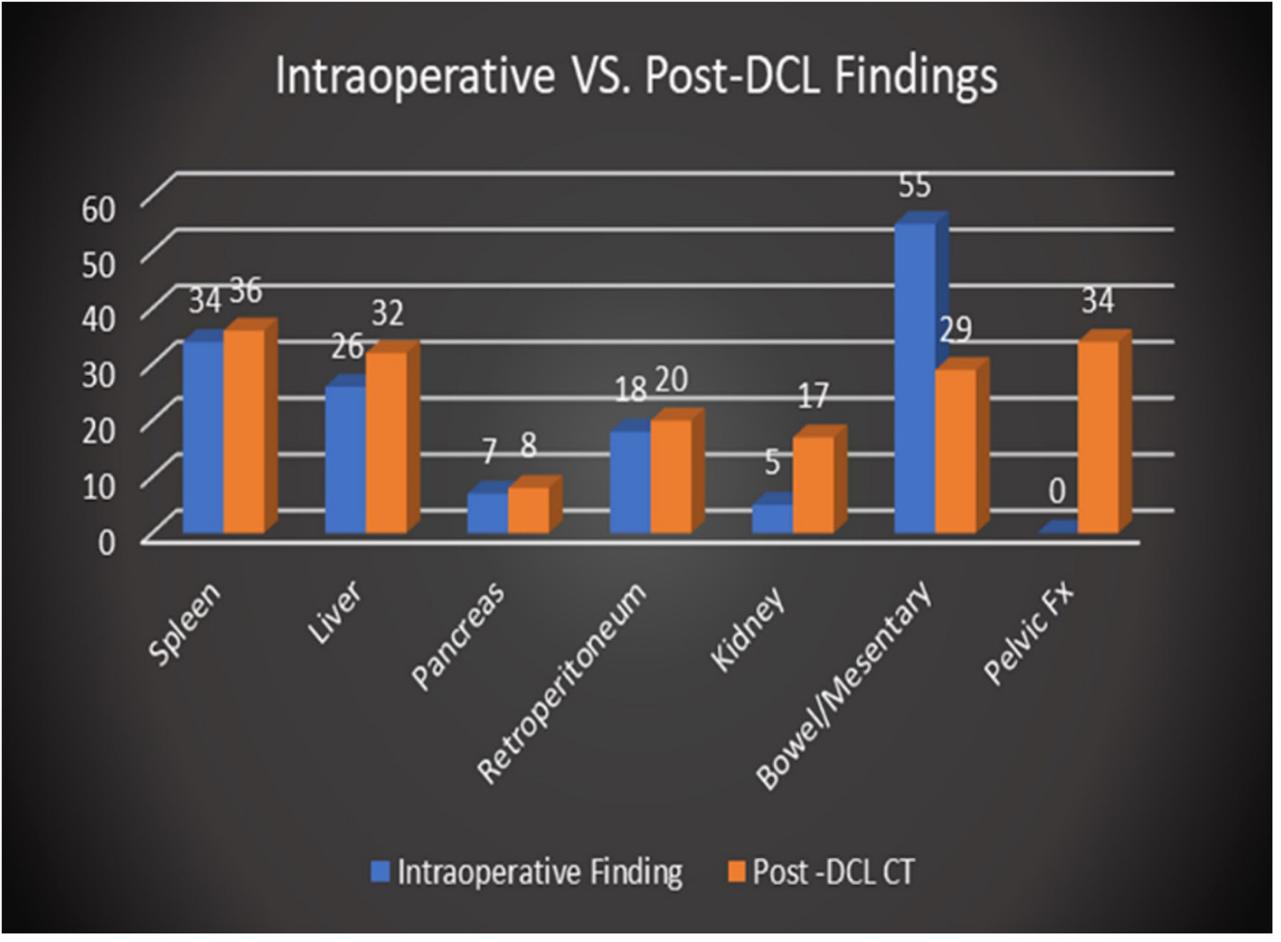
Comparison of overall distribution of injuries reported intraoperatively vs. on post-DCL CT.
